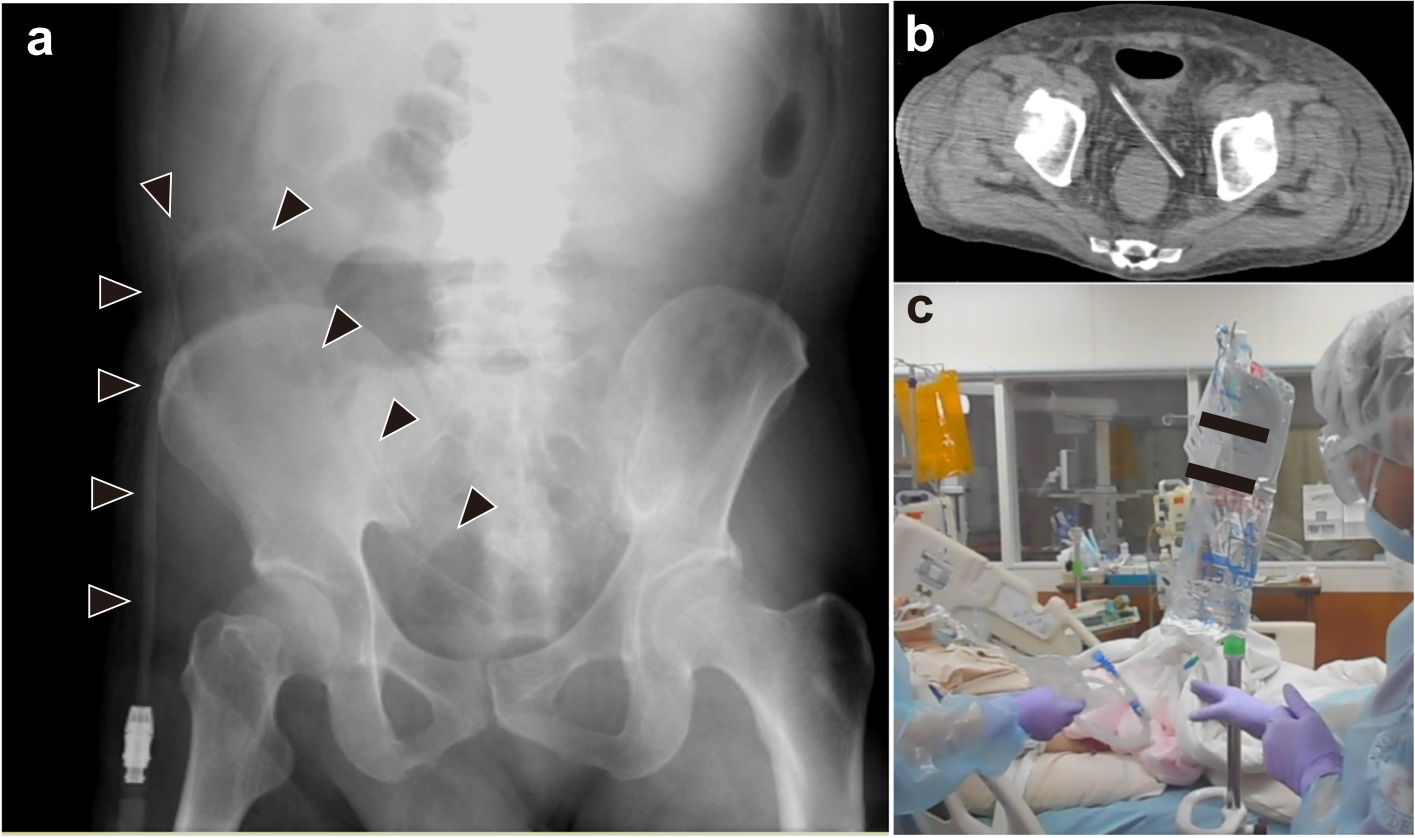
a Abdominal X-ray of peritoneal dialysis (PD) catheter. Arrowhead indicates the PD catheter. b The PD catheter tip confirmed in the recto-vesical pouch by CT scan image taken after ICU discharge. c Actual condition of peritoneal dialysis for COVID-19 intubated patients
